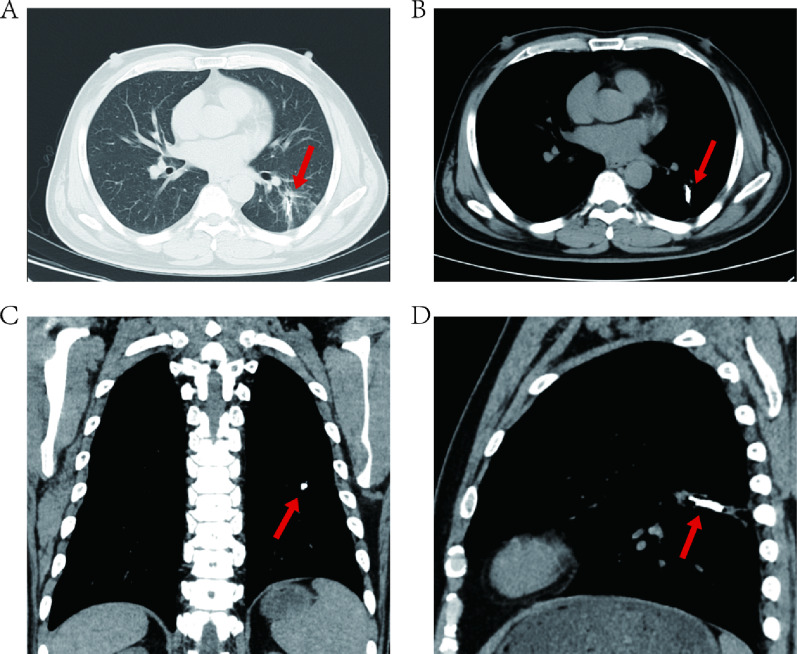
Chest CT images. (A) Axial lung window: a linear metallic density is seen in the basal segment of the left lower lobe, surrounded by patchy opacities. (B) Axial mediastinal window at the same level confirms the linear high-density foreign body. (C) Coronal and (D) sagittal reformatted images show the intrapulmonary location of the foreign body
